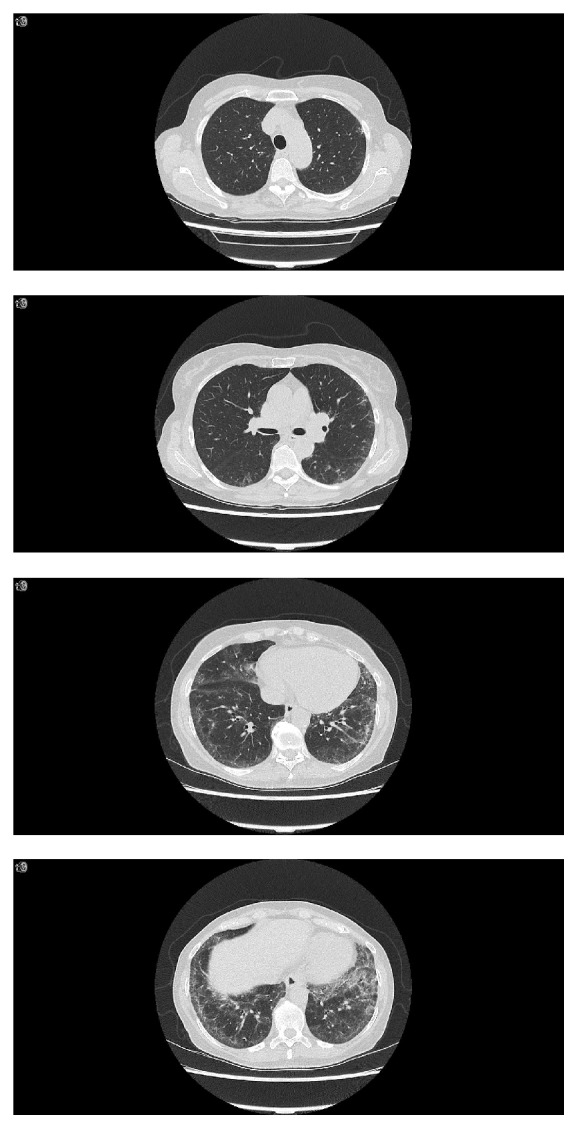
Chest computed tomography (CT) images showing lower lung zone predominant ground glass attenuation and reticulations present.
